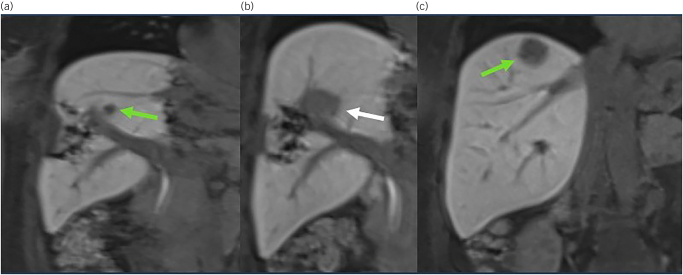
(a) Primovist MRI scan showing residual metastasis (green arrow) adjacent to right anterior portal inflow. (b) Primovist MRI scan showing SBAR defect (white arrow). (c) New metastasis in segment 7 (green arrow).
